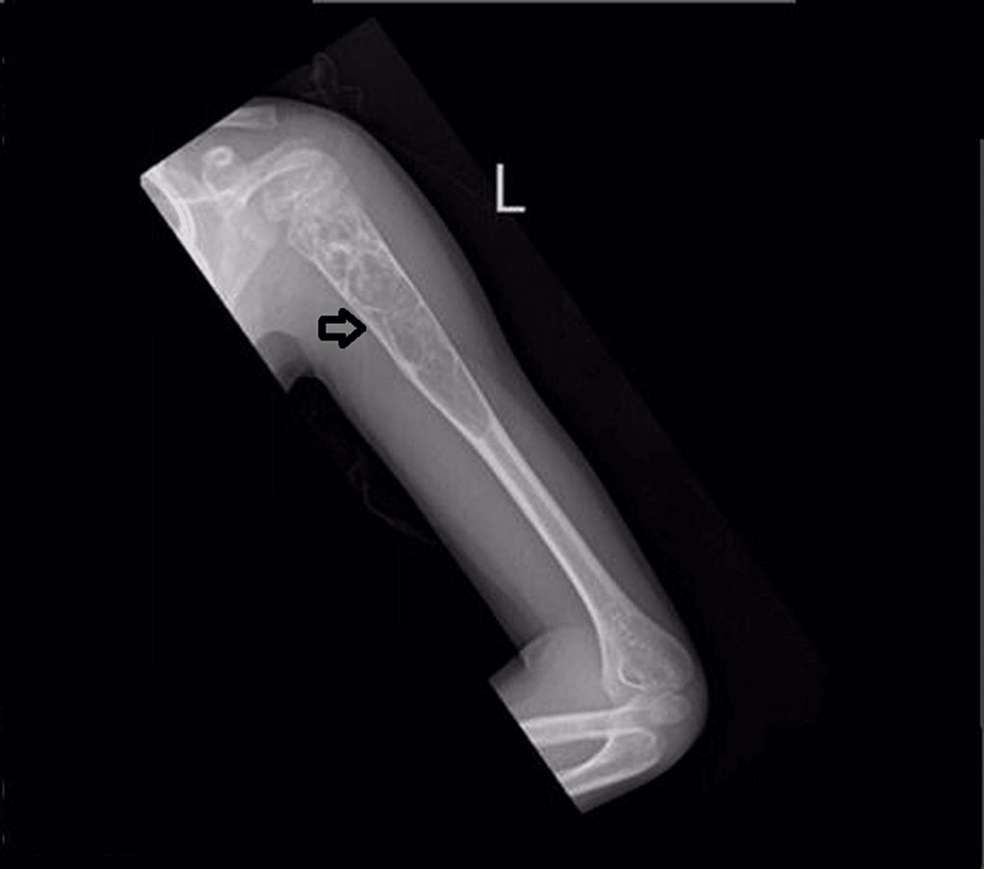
Plain X-ray of the left humerus Cystic lesion of the left upper humerus (arrow)
